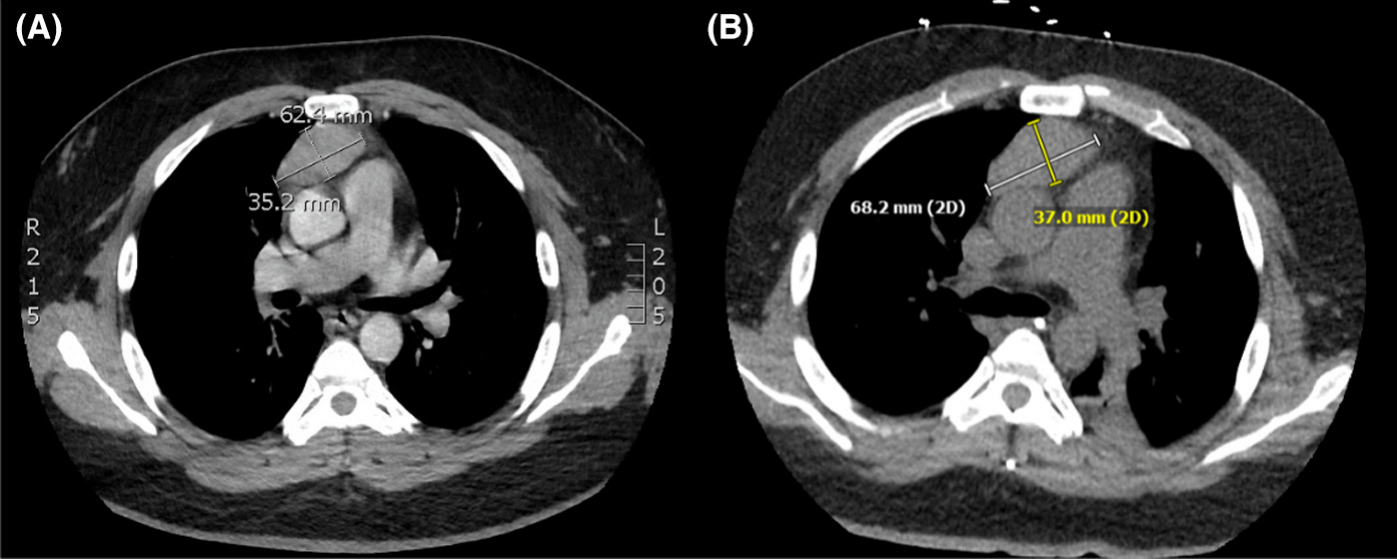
(A). Chest CT showing 6 × 3.5 cm anterior mediastinal mass consistent with thymoma 2 months prior to admission. (B). Chest CT showing anterior mediastinal mass which had increased in size to 3.7 × 6.8 cm.
